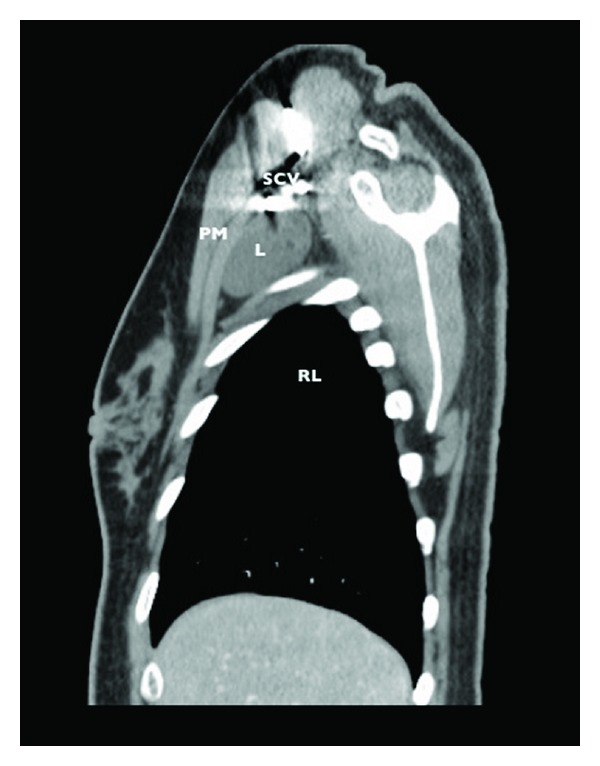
Chest CT with intravascular contrast, sagital view. PM: pectoral muscle; L: lymphocele; RL: right lung; SCV: subclavian vessels.
